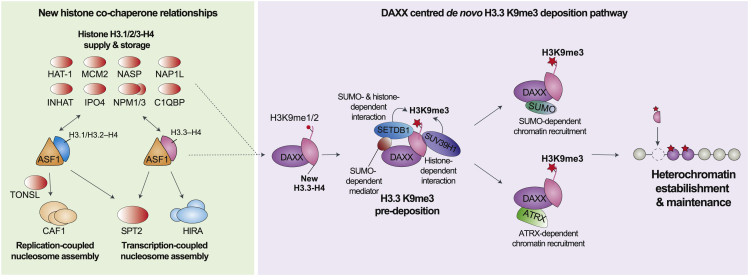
DAXX adds a *de novo* H3.3K9me3 deposition pathway to the histone chaperone network During histone supply ASF1 handles H3.1/2/3–H4 dimers and forms several histone-dependent co-chaperone complexes with other histone chaperones, notably ASF1 channels H3 variants toward distinct deposition complexes on chromatin. We identified a new ASF1-centered histone supply pathway to SPT2 that is H3 variant independent, as well as an upstream role for ASF1 in delivering H3.3 histones to DAXX, and an H3.1 variant specificity in TONSL. We found DAXX facilitates the catalysis of H3.3K9me3 through SETDB1 and SUV39H1 methyltransferase recruitment prior to histone deposition on chromatin. DAXX-bound H3.3–H4 recruits SETDB1 and SUV39H1, and the interaction of DAXX with SETDB1 is additionally dependent on SUMOylation. Other factors involved in heterochromatin establishment are differentially dependent on ATRX (e.g., the ChAHP complex) and SUMOylation (e.g., SMCHD1-LRIF1), and we speculate that this represents alternative pathways for H3K9me3 deposition supporting *de novo* heterochromatin silencing at distinct genomic locations.
